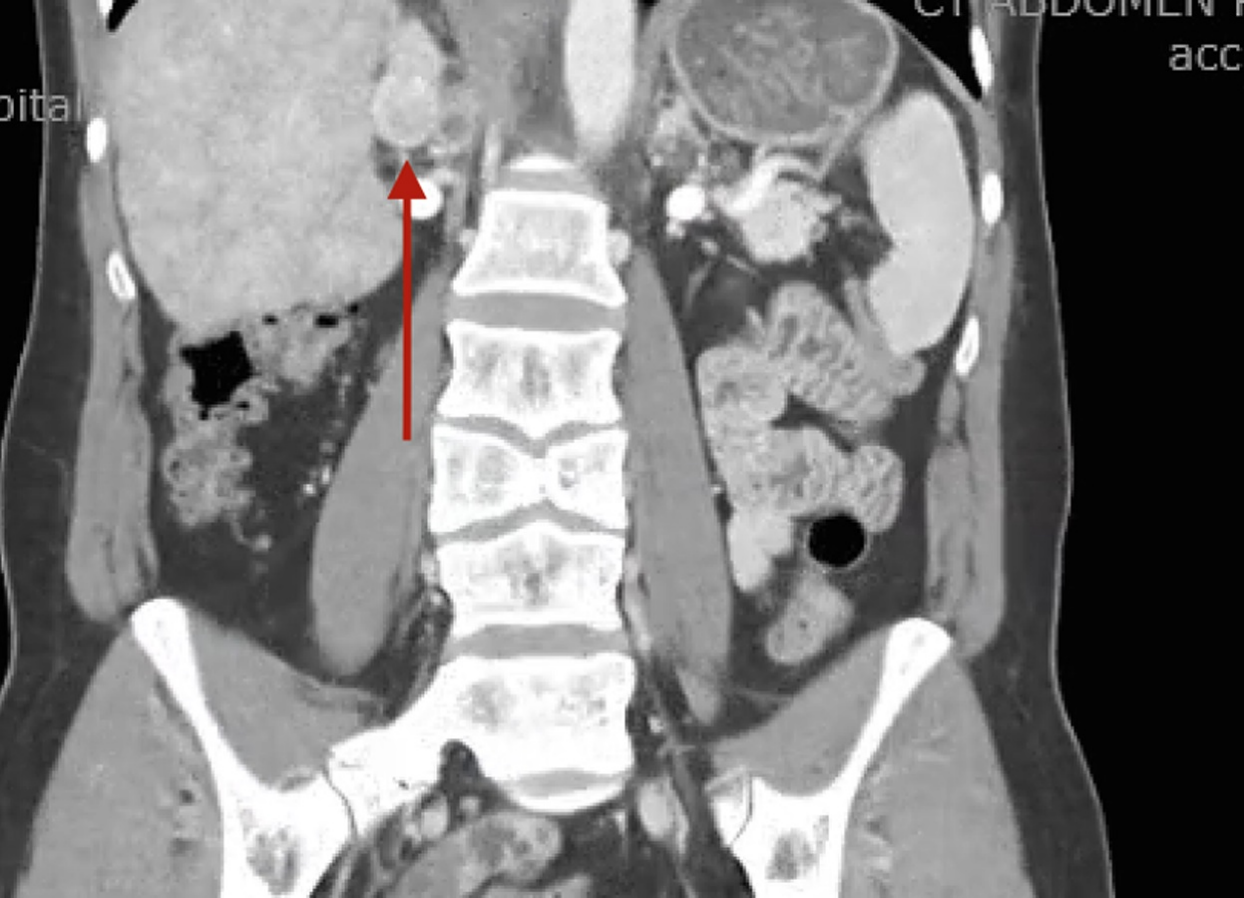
CT Scan Patient Two Red arrow shows large hypervascular right-sided adrenal mass
